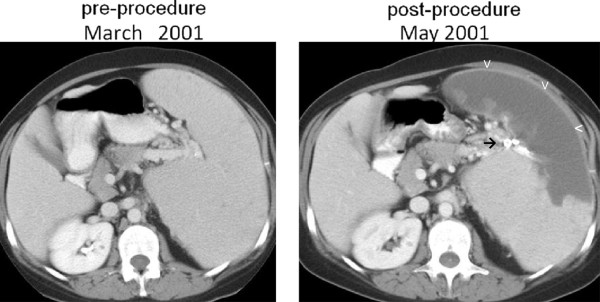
Patient 3, pre and post-procedure axial CT scans with contrast, demonstrating massive splenomegaly, the successful placement of radiopaque material in branches of the splenic artery (→), resulting in splenic infarction (v).
